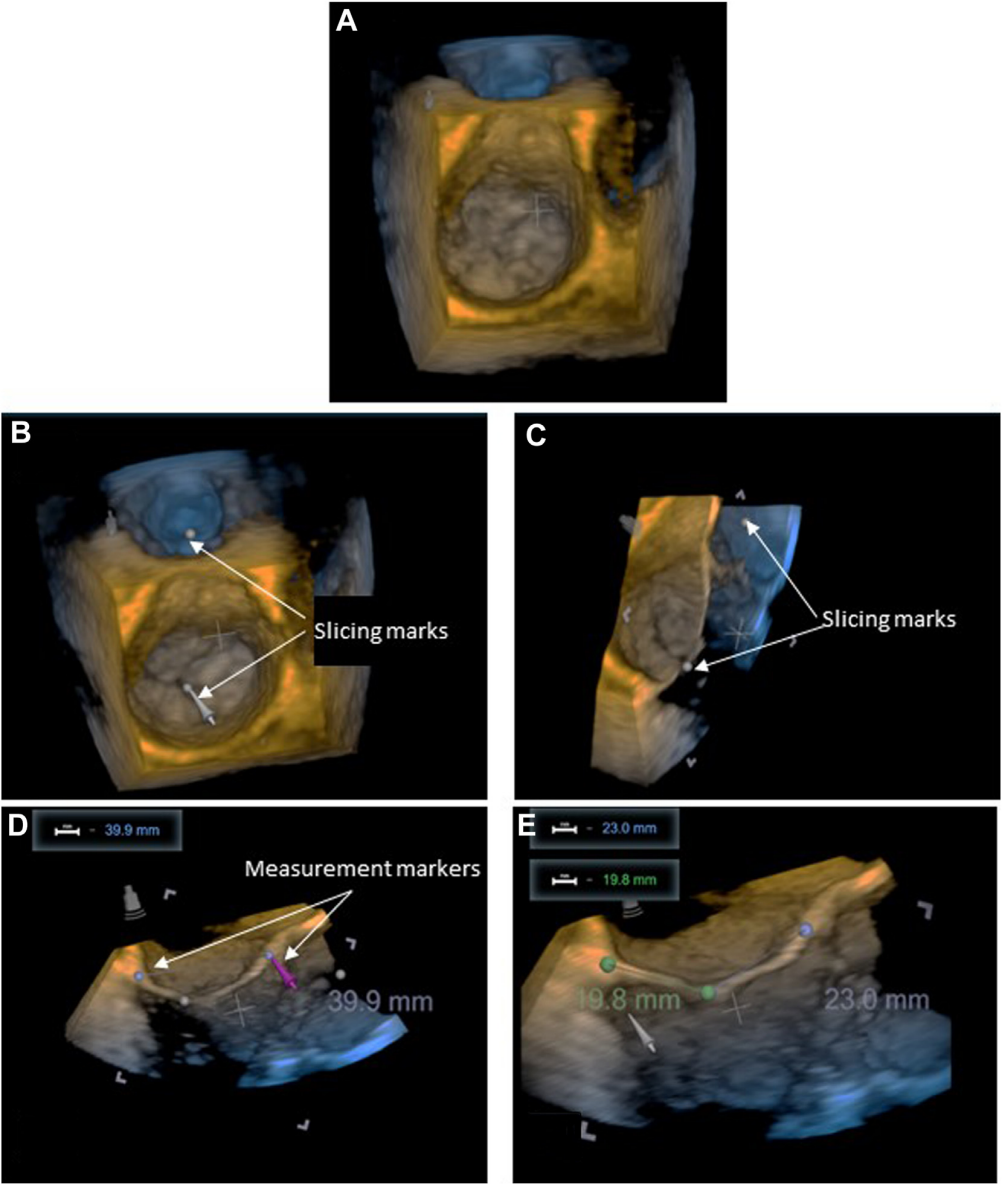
Main steps to measure mitral valve annulus and leaflets using the HOLOSCOPE-i (RealView Imaging, Yokneam, Israel). Pictures shown are as displayed on the 2-dimensional technician station, for explanation purposes only. (A) Transesophageal echocardiography–based full hologram, including the mitral valve. (B) anteroposterior (AP) plane marking; (C) AP slicing plane; and (D) AP linear measurement. (E) The anterior middle segment of mitral valve (A2), posterior middle sefment of mitral valve (P2) linear measurement.
